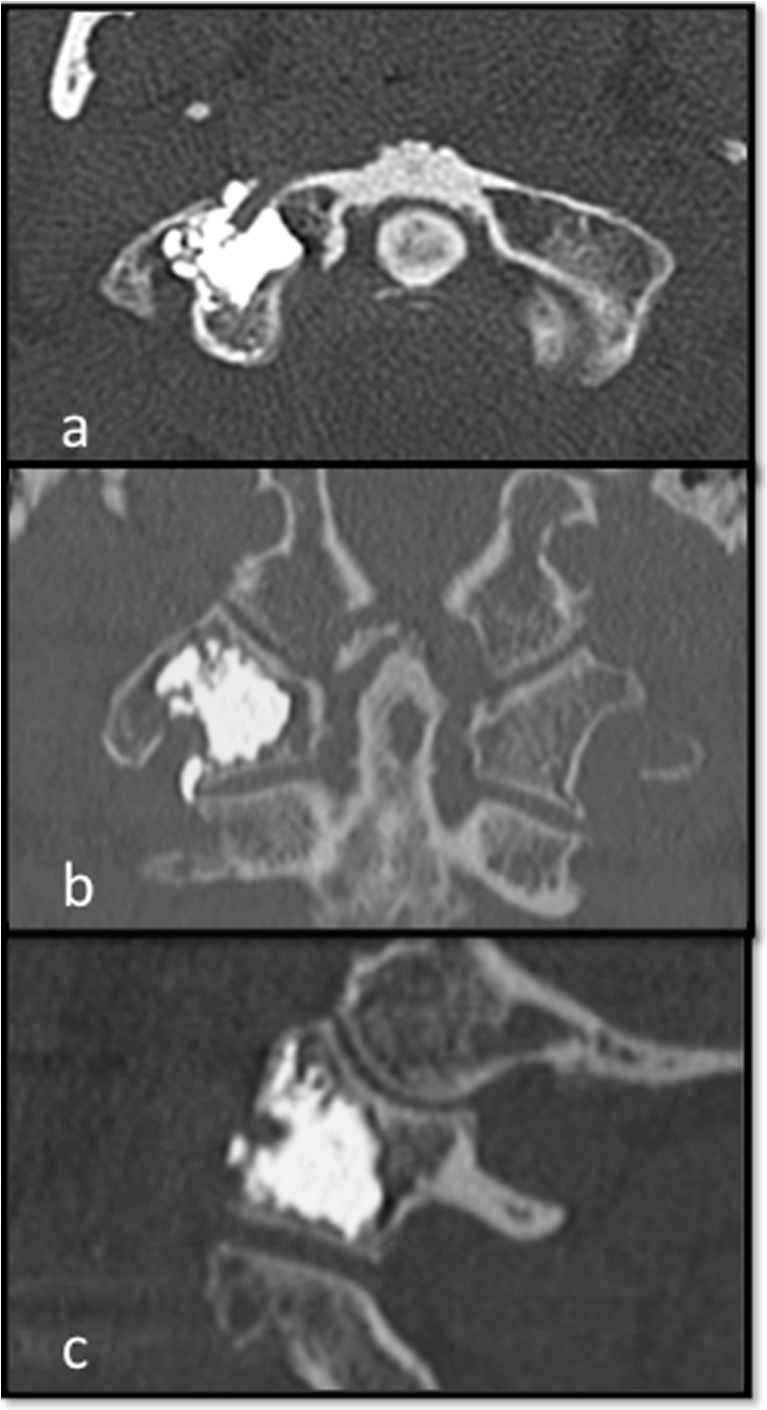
Intraoperative CT after cement augmentation [axial (a), coronal (b), sagittal (c)]
